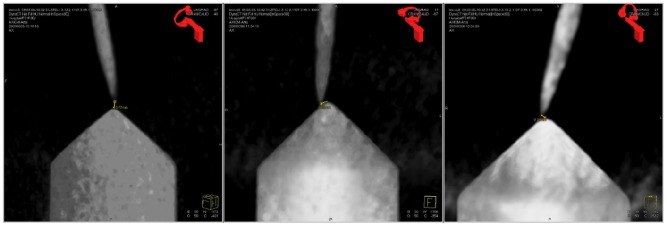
The error was defined as the distance between the needle's tip and the rod's tip measured on the reconstructed CT-images.
